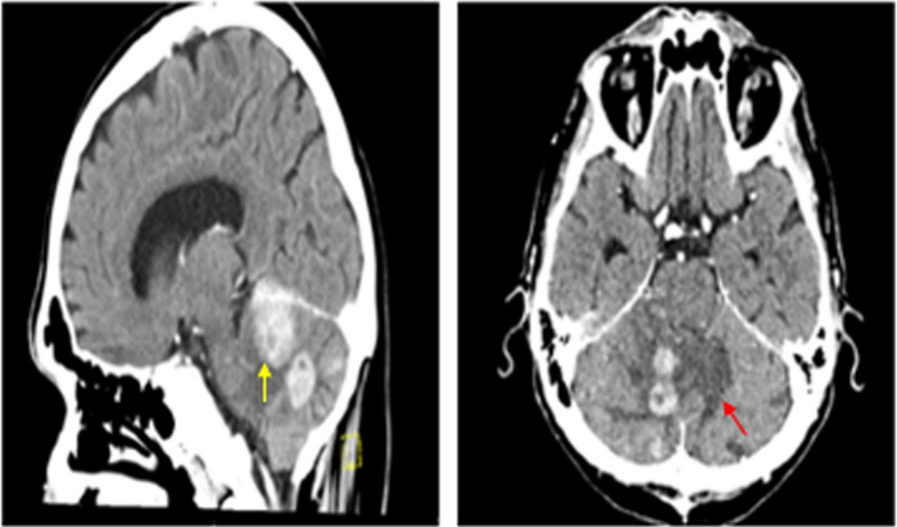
CT with contrast showing enhancing cerebellar lesion (yellow arrow) and vasogenic edema (red arrow)
